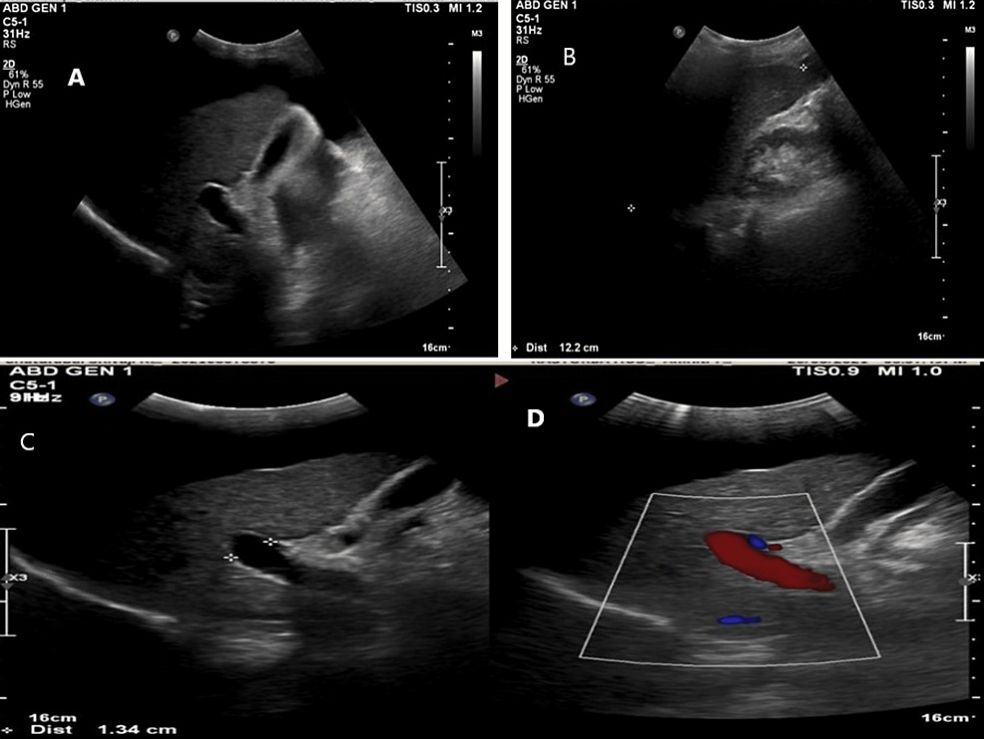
Ultrasonography of the abdomen and pelvis of the patient showed (A) a normal gallbladder with no dilatation; (B) evidence of a small-sized (9.7 cm), shrunken liver with coarsened echotexture of parenchyma and irregular margins with a normal biliary tree; (C) portal vein dilation (12 mm); (D) normal blood flow in the portal vein on color doppler imaging.
